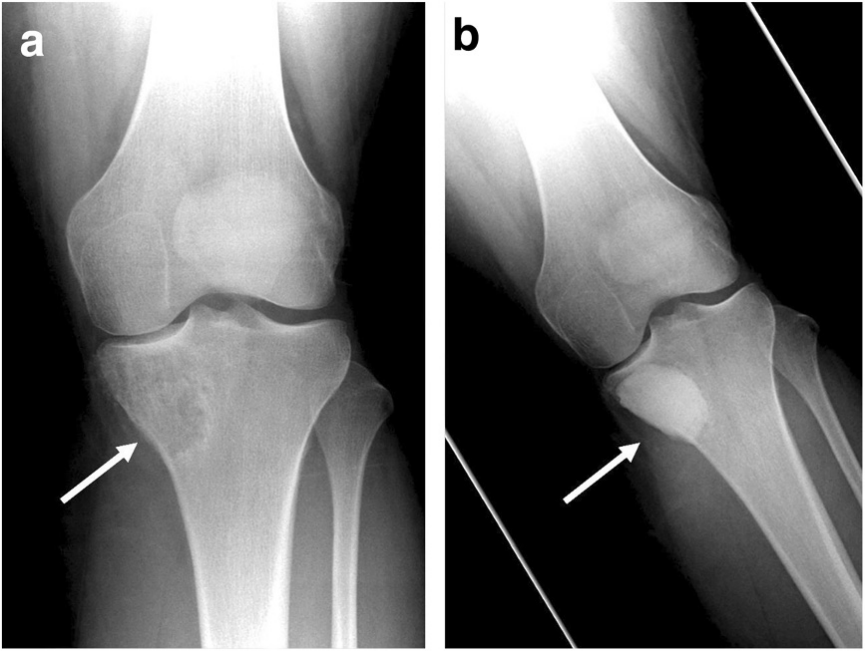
Radiographs showing CMF of the tibia with postoperative appearances following curettage and cementoma. (a) Anterior-posterior radiograph of the left knee showing a lytic lesion (arrow) in the medial tibial plateau with a thin, partially sclerotic margin and very mild bone expansion. There is no appreciable matrix mineralisation, and the articular surface of the knee joint is well-preserved. (b) Anterior-posterior radiograph of the left knee following curettage and cementation (arrow), showing satisfactory post-operative appearance.
